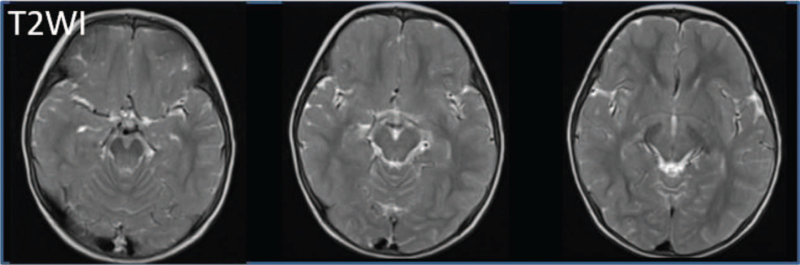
Follow-up MRI performed on 1 yr and 8 mo old shows symmetric high signal intensity changes in the superior cerebellar peduncle, lateral pons, thalami, posterior limb of internal capsule on coronal T2-weighted image. MRI = magnetic resonance imaging.
